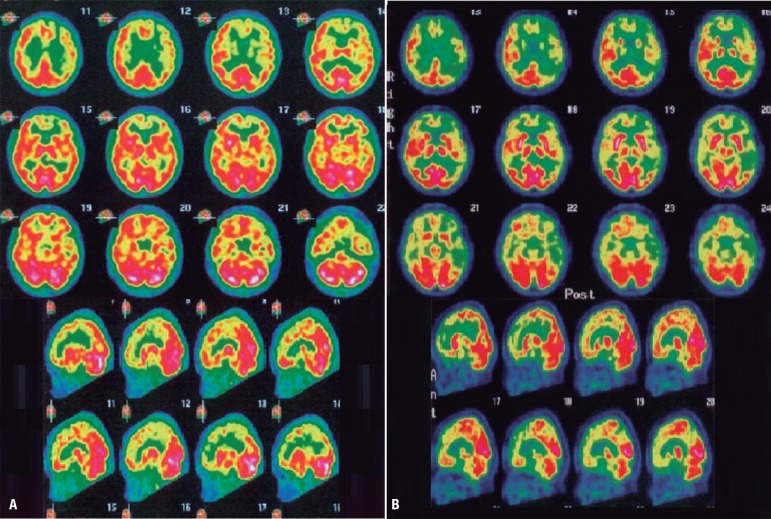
Brain scintigraphy (SPECT) from patient TA, 48 year-old woman. [A] Brain
